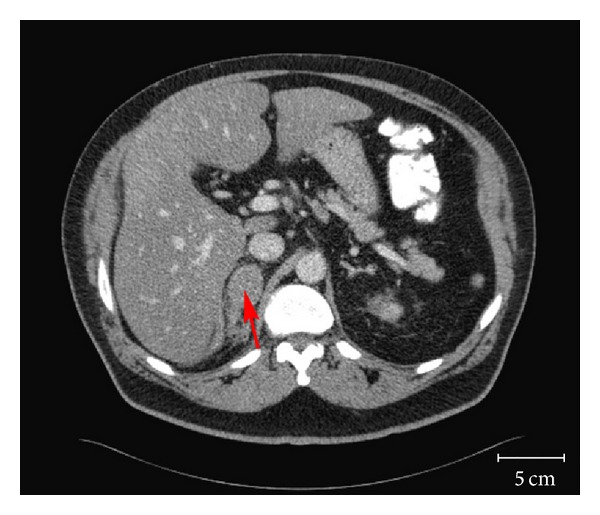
Abdominal computed tomography (CT) scan revealing a 3.6 × 2.7 cm mass (red arrow) involving the right adrenal gland.
